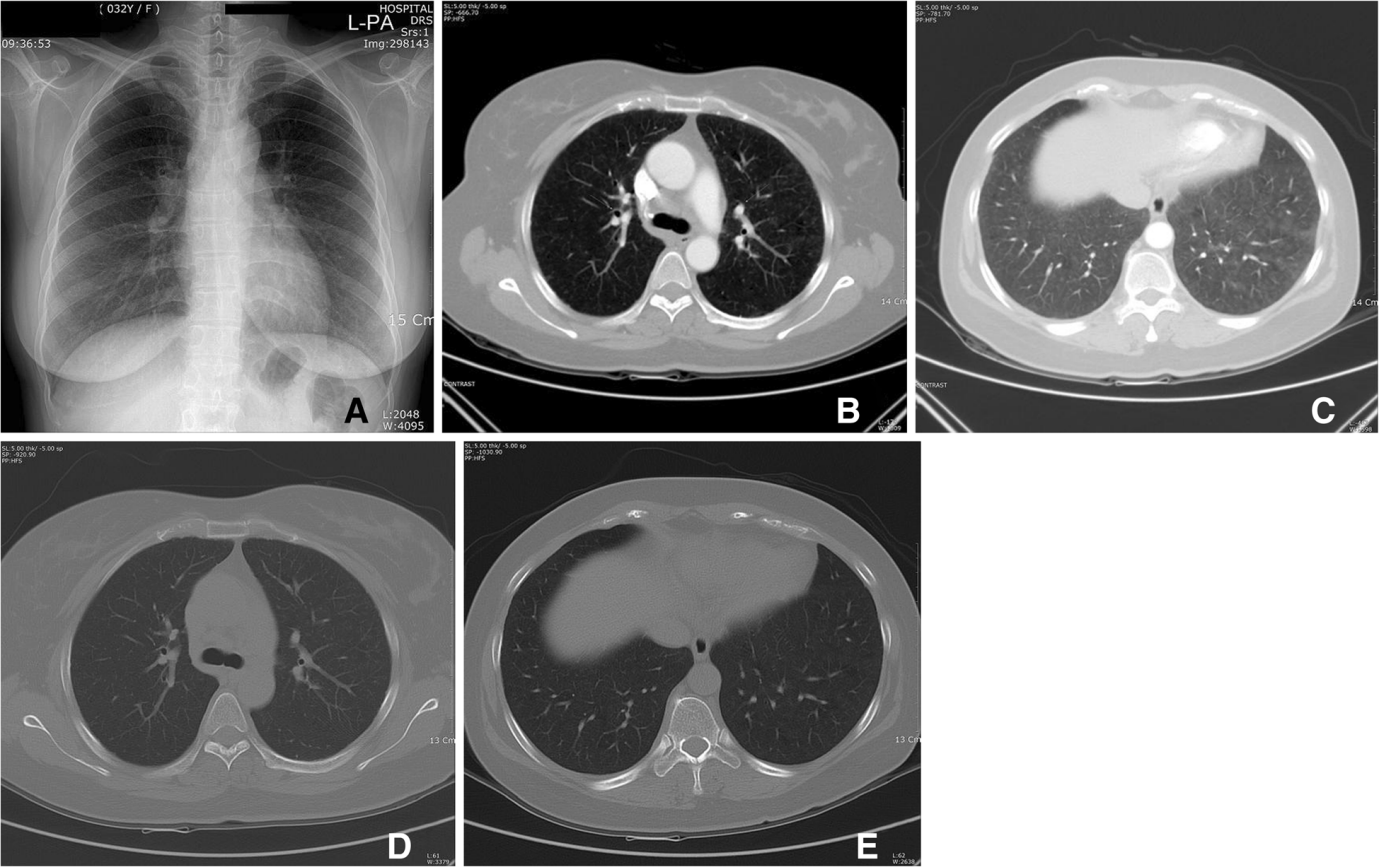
Image findings. A. A simple chest radiograph shows no active lesions in either lung. B. Chest computed tomography (CT) at the time of diagnosis shows mildly enlarged lymph nodes (arrows) in the mediastinum and both hilar and interlobar areas. C. The same chest CT also shows diffuse centrilobular ground-glass nodules in both lungs. D. Mildly enlarged lymph nodes in mediastinum and both hilar and interlobar areas can be observed on chest CT taken after being treated with medical intervention and avoiding occupational dust from the workplace. E. The density of diffuse centrilobular ground-glass nodules is decreased in both lungs after being treated with conservative measures.
